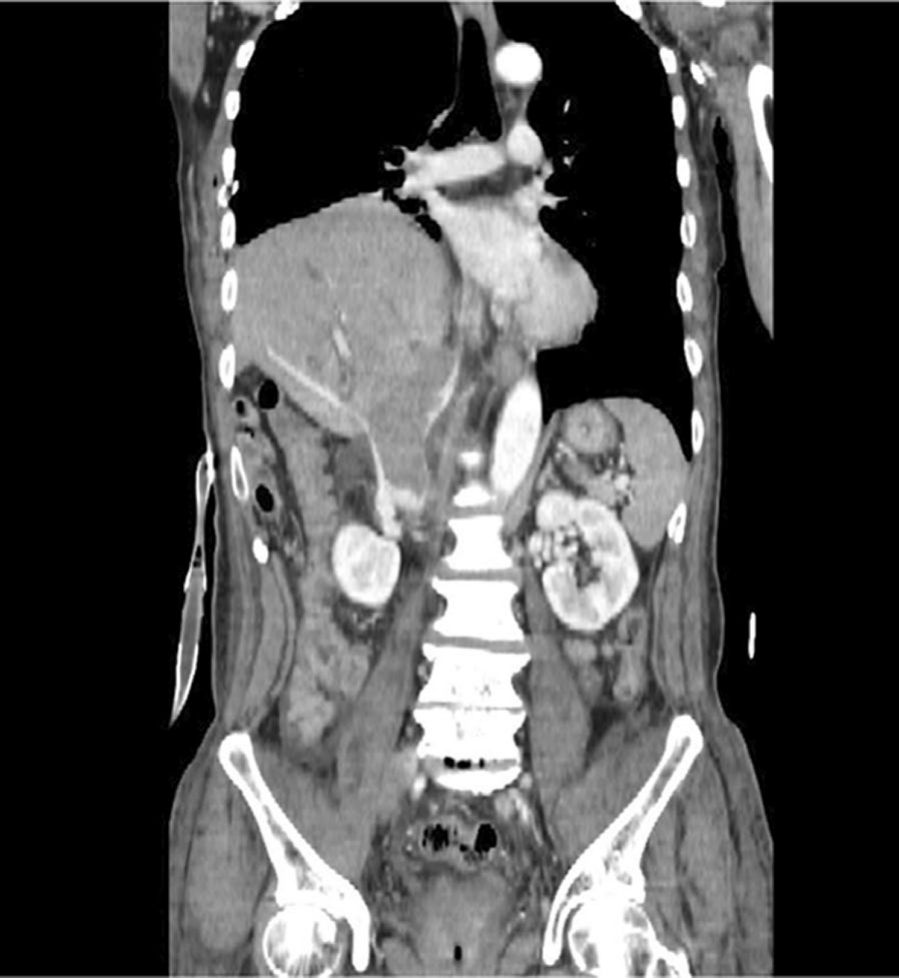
Follow-up enhanced CT scan findings on the ninth day of admission. Compared to the CT scan on the admission, the liver was more elevated, and a diagnosis of traumatic diaphragmatic injury was made. The diaphragmatic hernia caused severe narrowing of the inferior hepatic vena cava and formed a 13 cm × 3 cm giant thrombus in the inferior vena cava
